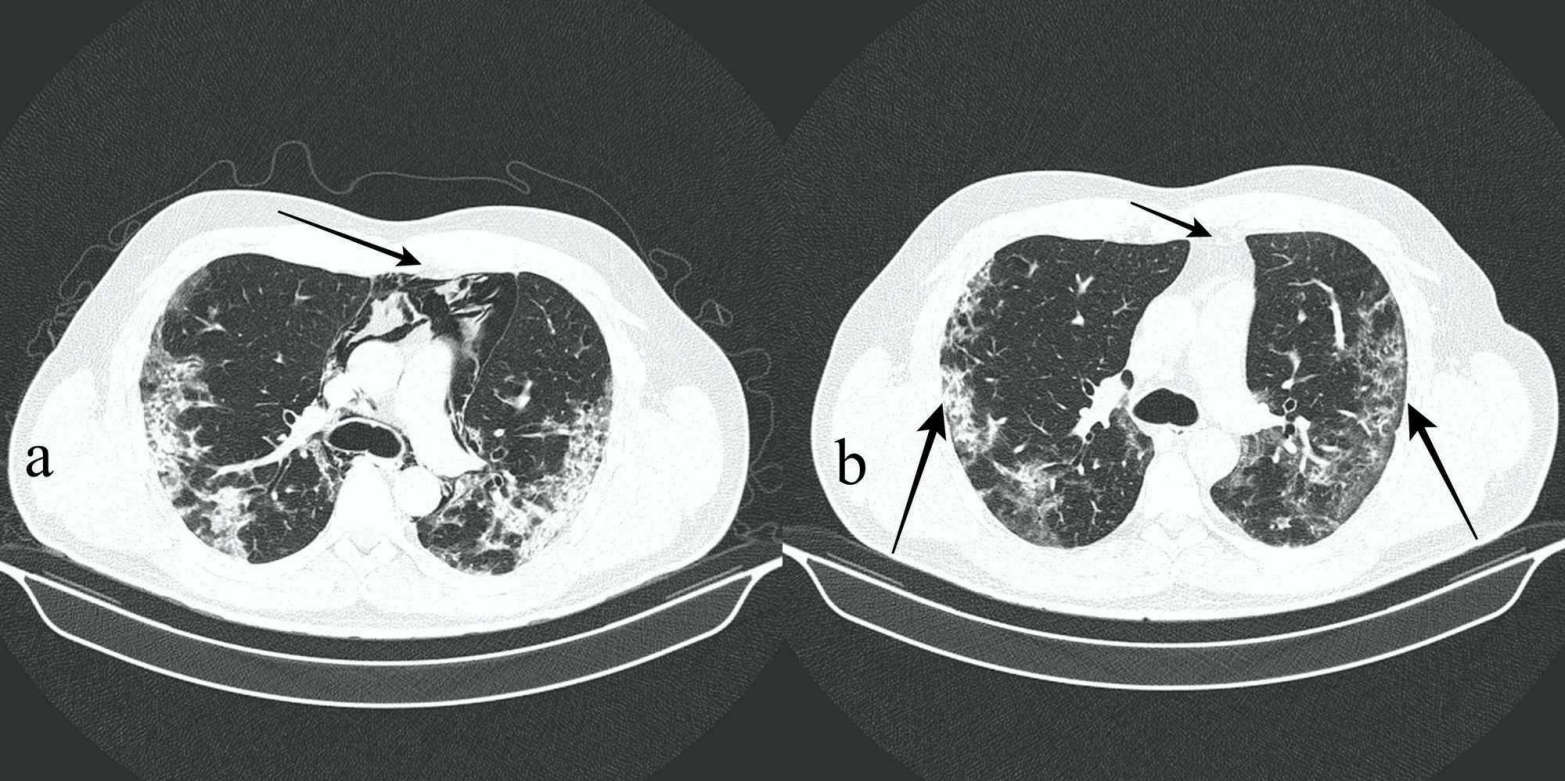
(a,b) Axial HRCT images demonstrating full resolution of spontaneous pneumomediastinum after one month since initial acute symptoms (b, short arrow). However, residual mixed attenuation bilateral and peripheral predominant opacities were still present (b, long arrows). See significant volume pneumomediastinum in the third week of disease for comparison (a, long arrow). HRCT, high-resolution CT
